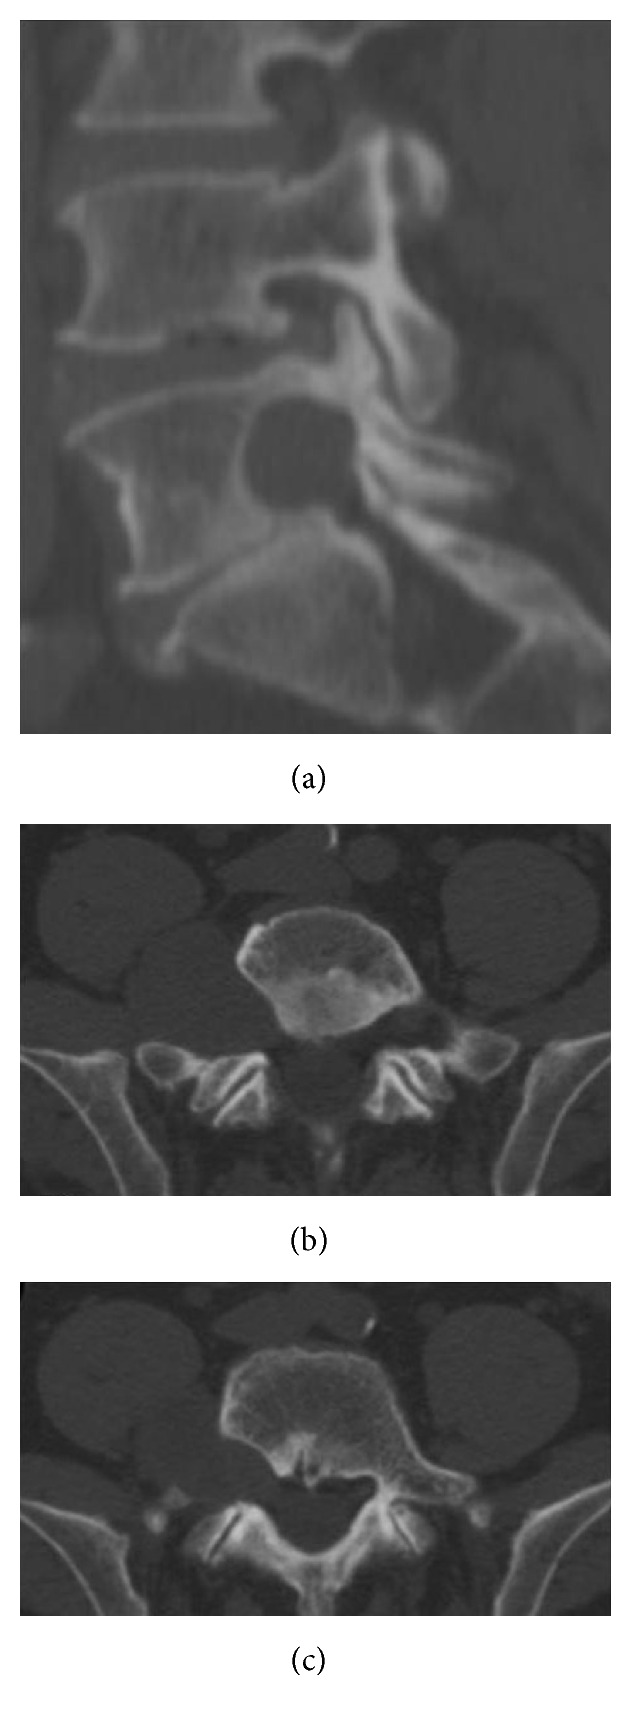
Preoperative spinal CT scan without contrast. The mass widened the omolateral conjugate foramen (a) and extended to the sacrum and to the ipsilateral sacroiliac joint eroding the right posterolateral portion of the L5 vertebral body (b, c).
